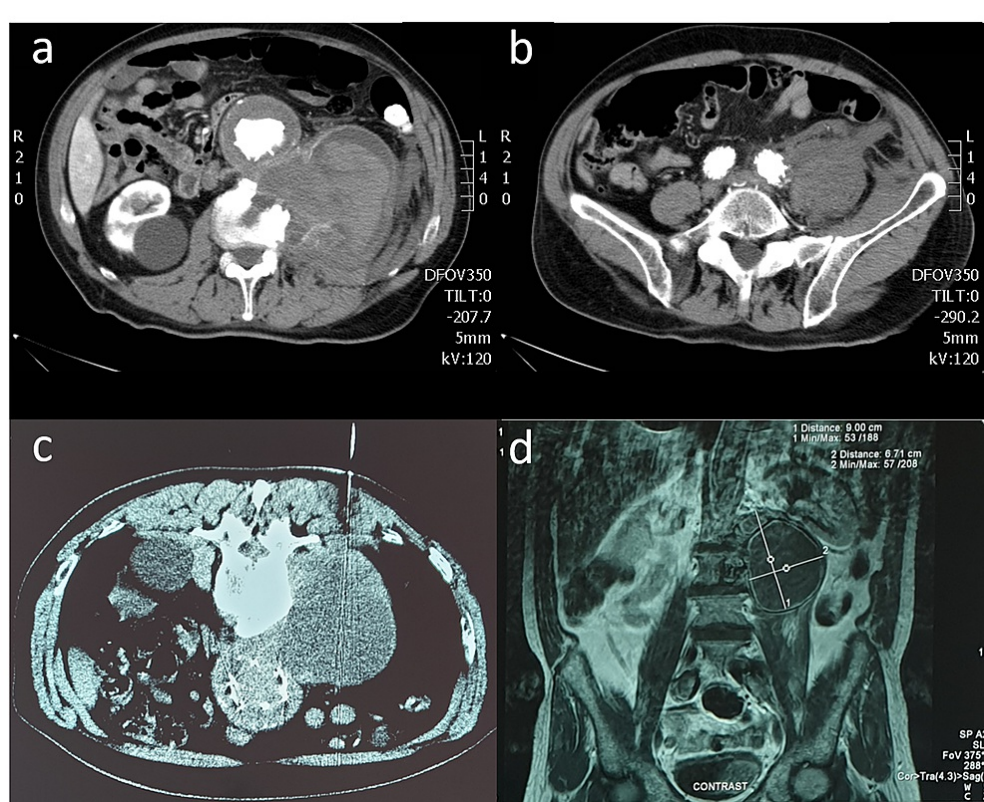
Pre-operative imaging workout of the patient (CT-scan, CT-guided core needle biopsy and MRI-scan) a & b: CT-scans, contrast enhanced, axial views. Notice the eroded body of the L2 vertebra and the large retroperitoneal mass being completely separated by the aneurysmatic sac (a) and the communication between the aneurysmatic sac of the left iliac branch junction of the stent and the retroperitoneal tumor at the L5-S1 level, depicting the rupture of the aneurysmatic sac (b). c: Computed tomography guided core-needle biopsy of the mass. Based on the biopsy track, the sample taken originated from the upper part of the mass, hence the aneurysmatic sac was not compromised at that level d: MRI-scan, contrast enhanced T2 weighted image, coronal view, depicting the large retroperitoneal mass being in direct contact with the L2 and L3 vertebrae that have signs of erosion.
